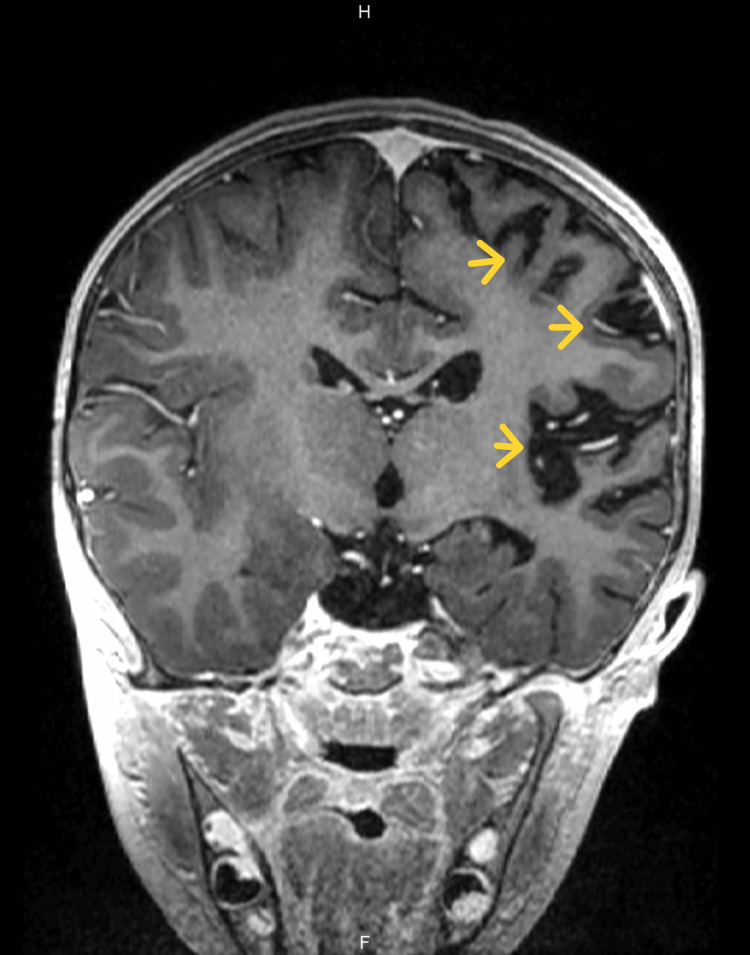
Second brain MRI, contrasted study, coronal section This figure details marked compromise of the insular region of left hemisphere also observed in the first brain MRI of the patient.
